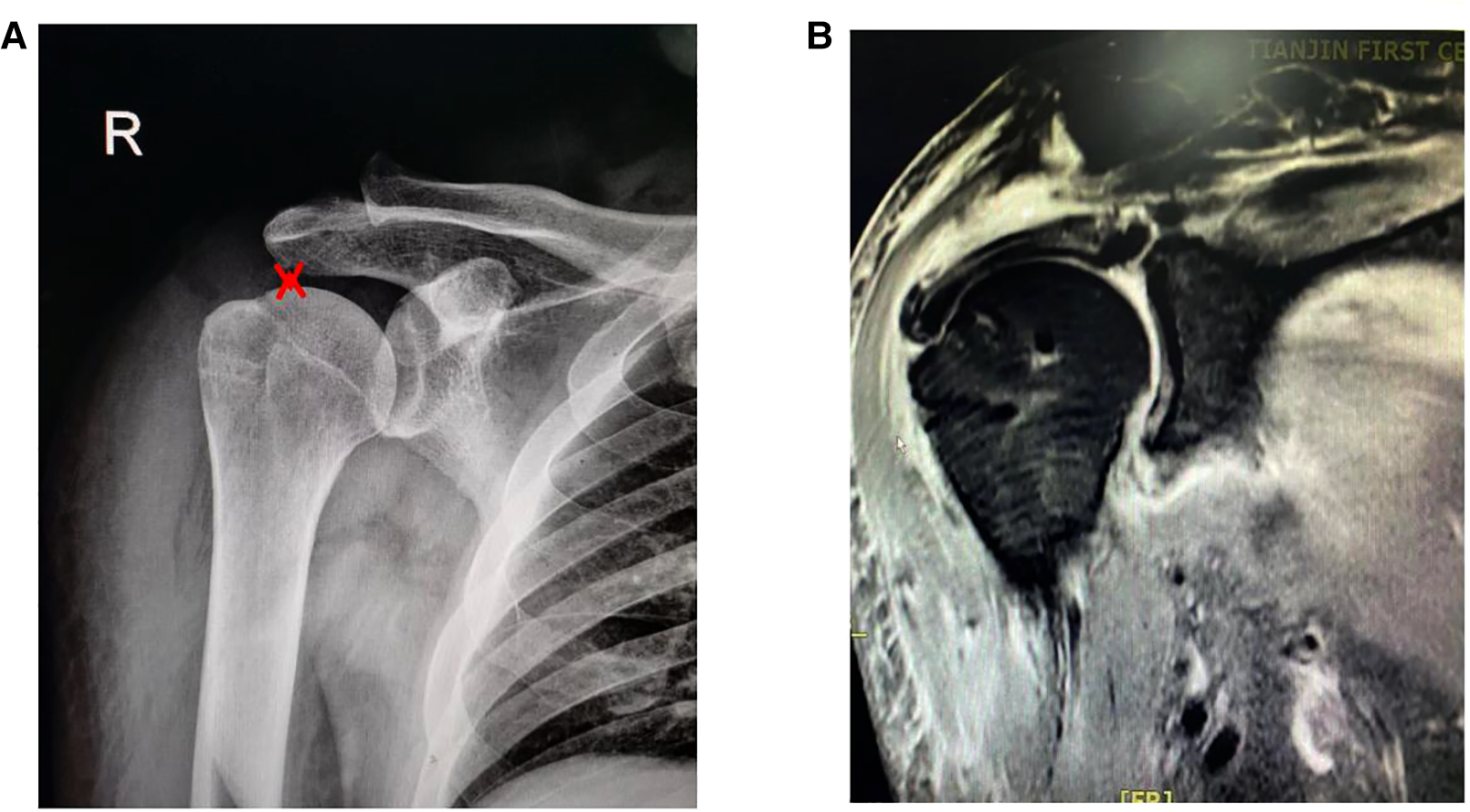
Postoperative radiograph of arthroscopic superior capsule reconstruction (1 month). (A) Red mark on the X-ray shows that the AHD is 9.5 mm; (B) the fascia lata medial side attached to the superior glenoid at 10–11 o'clock and 11–12 o'clock positions in the right shoulder, using two fully-threaded titanium suture anchors (diameter, 5 mm; suture anchor, Arthrex).
